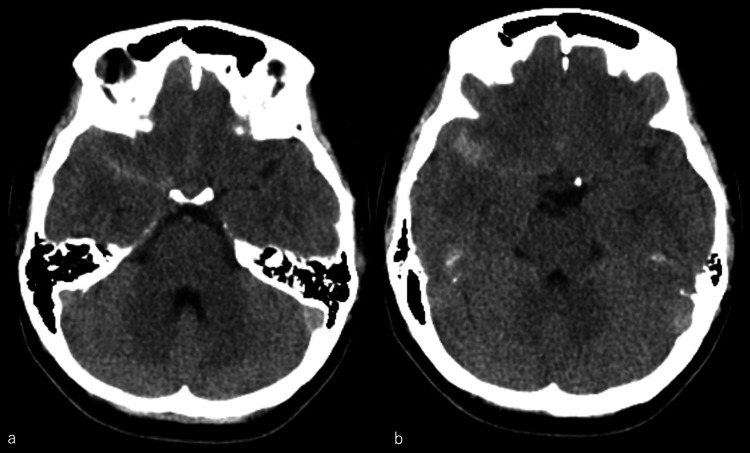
a, b) Head CT revealing a subarachnoid hemorrhage almost localized to the right Sylvian fissure where the plexiform arterial network was observed.
